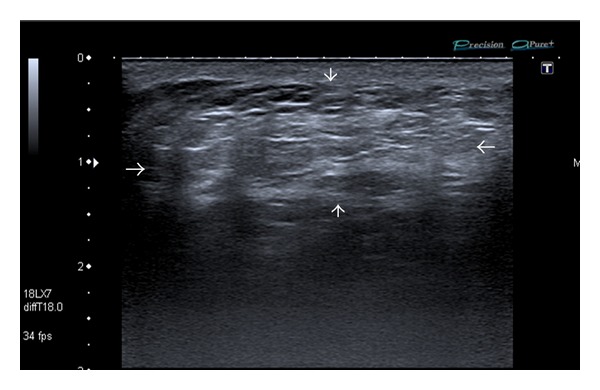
Ultrasound examination (longitudinal view) demonstrates a large subcutaneous mass (bounded by white arrows) in the interscapular region with sonographic features consistent with a lipoid mass, that is, elliptical shape, heterogeneity, longest axis parallel to the skin surface, lack of posterior acoustic enhancement or attenuation, and presence of multiple echogenic lines perpendicular to the ultrasound beam.
